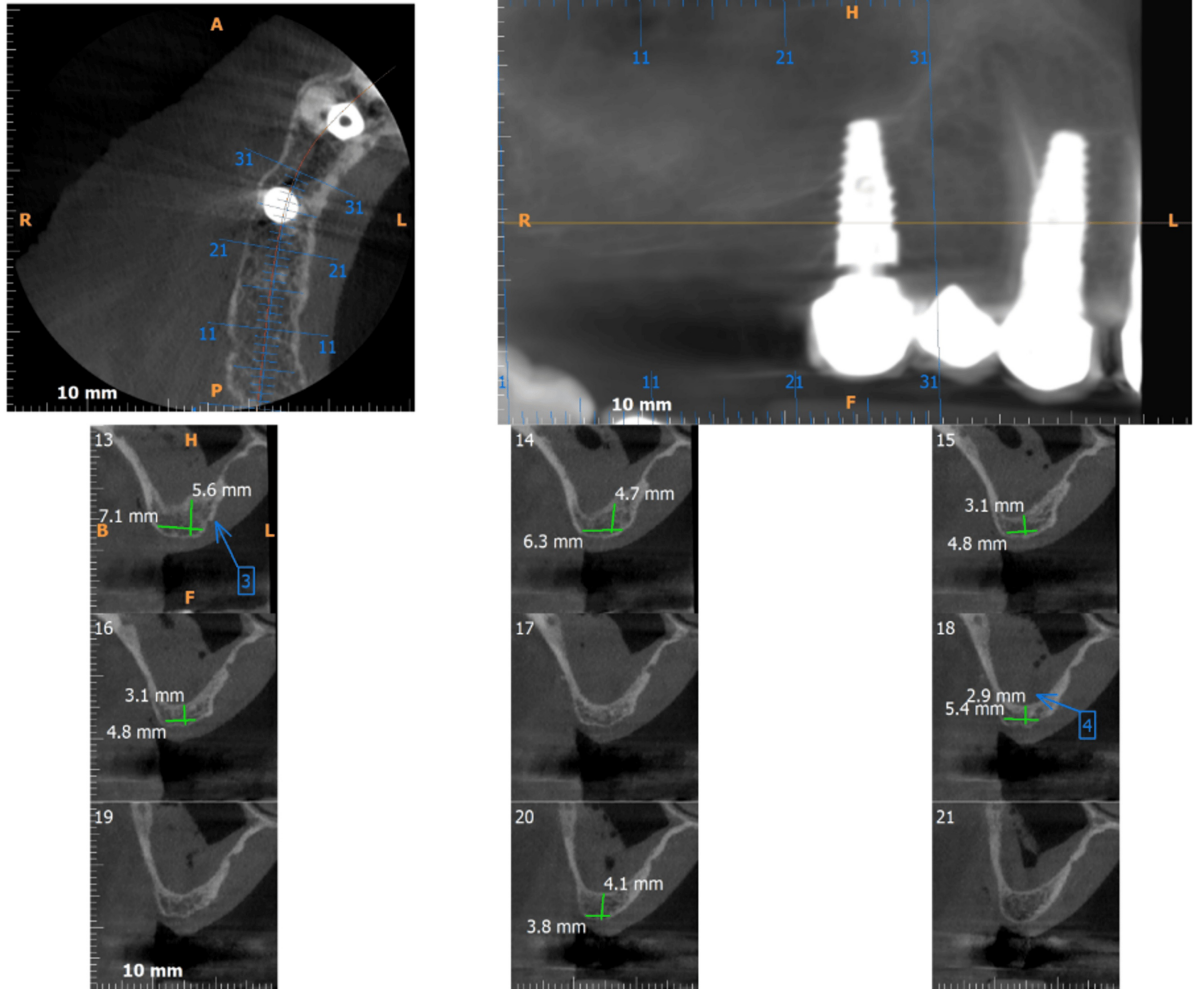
Preoperative cone beam computed tomography showing reduced residual alveolar bone height in region 16.
